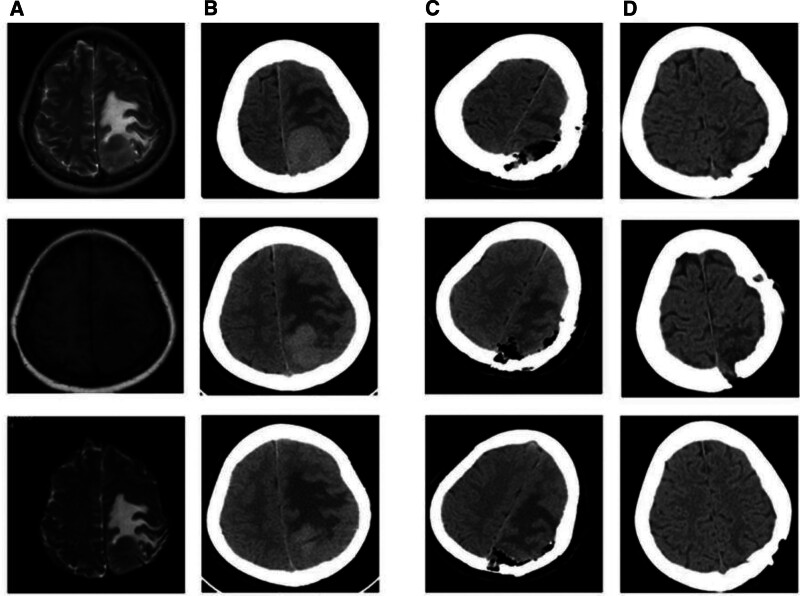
The imaging studies conducted for the patient include: (A) preoperative MRI on December 28, 2023. (B) Preoperative CT on January 2, 2024. (C) Postoperative CT on January 3, 2024. (D) Postoperative CT on March 14, 2024.
